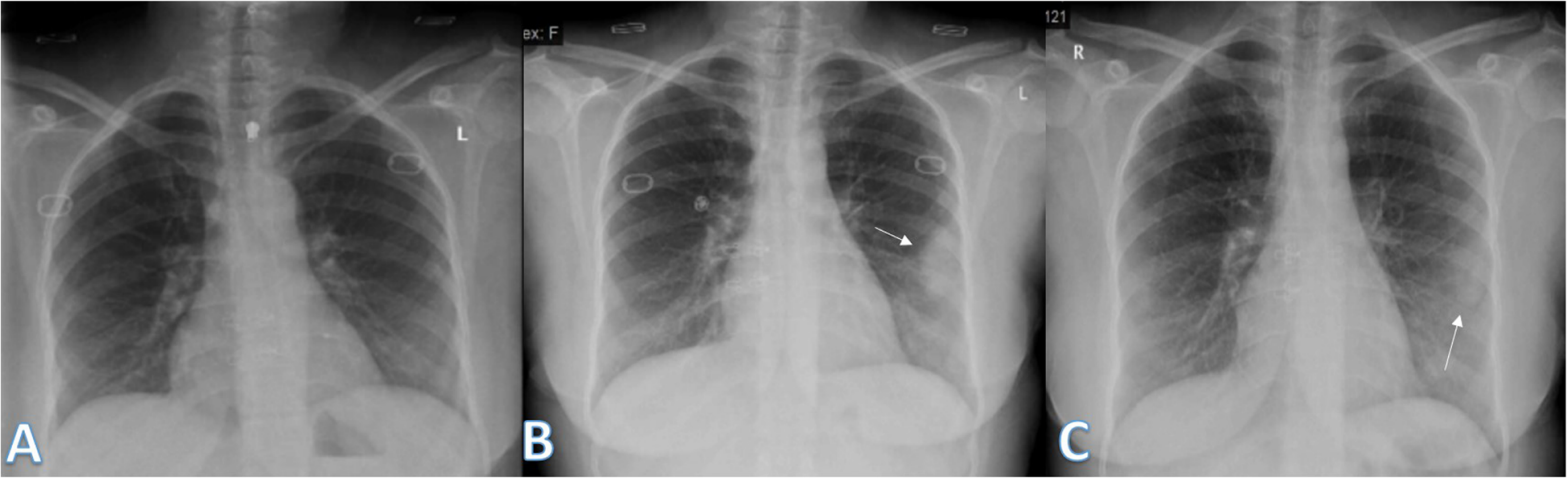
41-year female with positive COVID-19 virus. Initial chest X-ray (a) was normal. On the 3rd day, follow-up chest X-ray (b) shows a well-defined large solitary pulmonary nodule (arrow) at the periphery of the left middle lung zone with TSS as 1. On the 18th day (c), the lesion was partially resolved with faint density (arrow)
